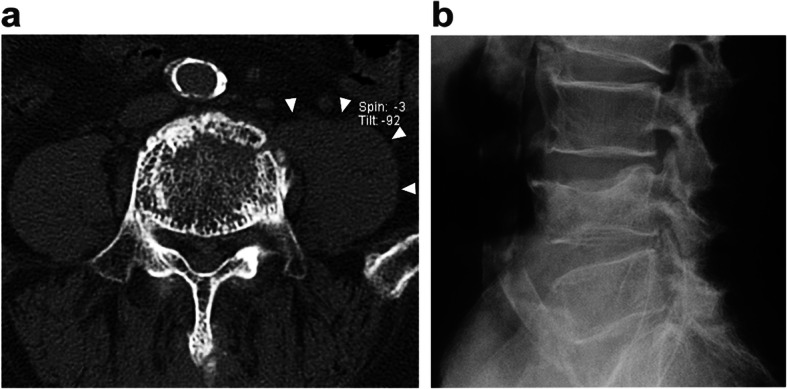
Follow-up computed tomography and X-ray image. a Plain computed tomography on 2 months after the patient’s hospital admission shows normal size of the psoas major muscle (arrowhead), which indicates disappearance of the hematoma. b X-ray at final follow-up 1 year after injury revealed bony union with minimal deformation
